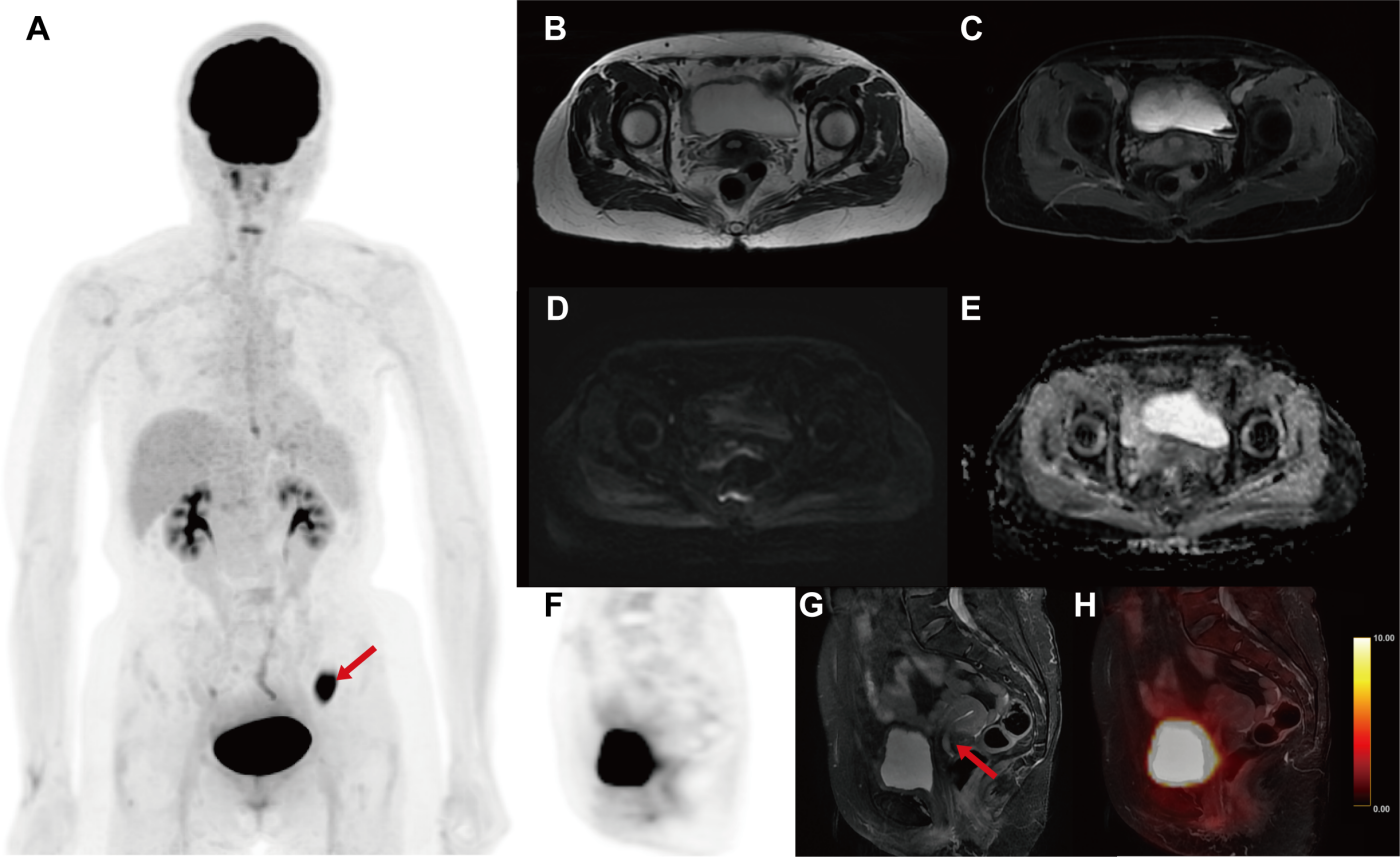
The multimodal 18F-FDG PET/MR images for evaluating the uterine. (A) MIP image of whole-body PET. (B) Axial T2WI. (C) Axial T1WI+C. (D) Axial DWI. (E) Axial apparent diffusion image (ADC) map. (F) Sagittal PET image. (G) Sagittal fs T2WI. (H) Sagittal PET/T2WI fusion image. On the pelvic sagittal MRI, there was a polypoid mass in the lower segment of the uterine (red arrow). The polypoid lesion showed a slightly high intensity signal on T1WI and fs T2WI, but no restricted diffusion signal in DWI and ADC. A mild enhancement of the lesion was observed on T1WI+C. The PET and PET/MR fusion images showed slight hypermetabolism in the lesion (SUV_max_ = 5.7g/ml). Unexpectedly, the MIP image of whole-body PET revealed an abnormal hypermetabolism in the left lower abdomen (red arrow, SUV_max_ = 17.68 g/ml).
